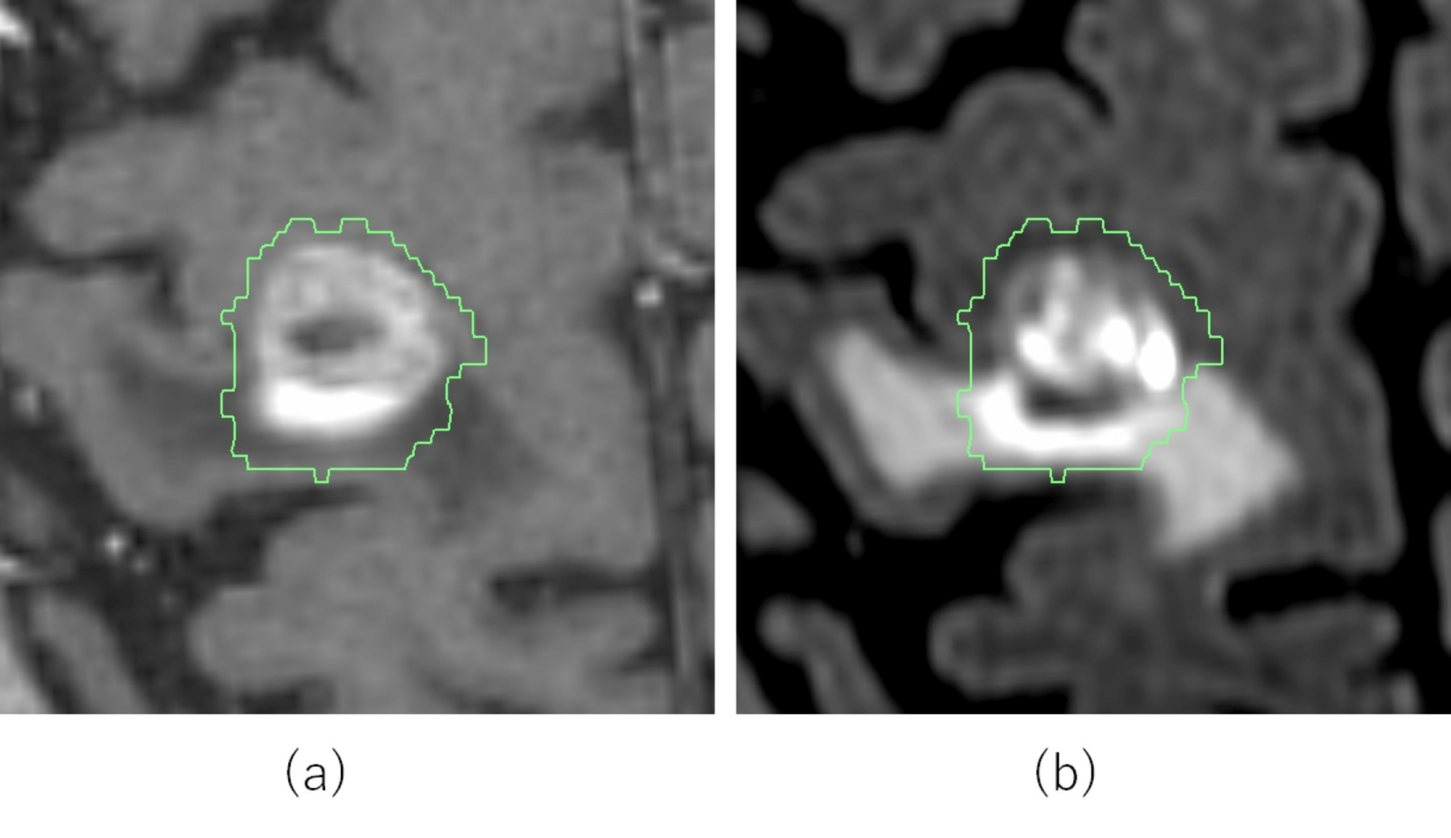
Delineation of gross tumor volume by jointly referring to (a) thin-slice contrast-enhanced 3D T1-weighted (CE-3D-T1W) and (b) thin-slice contrast-enhanced 3D fluid-attenuated inversion recovery (CE-3D-FLAIR) magnetic resonance images.
